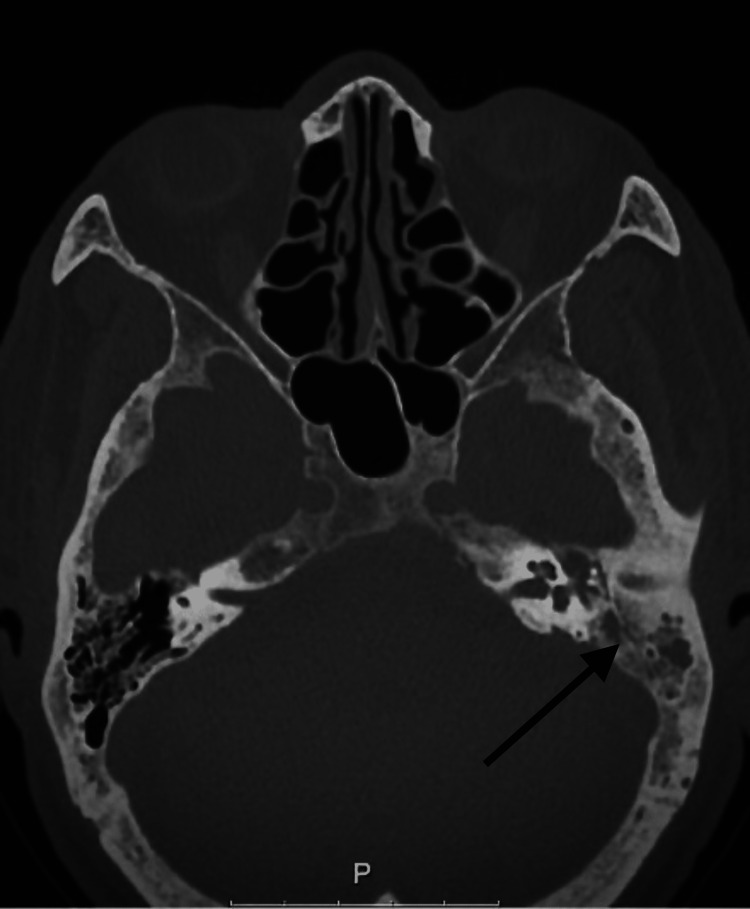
Ear CT revealing skull base osteomyelitis and left otomastoiditis.
